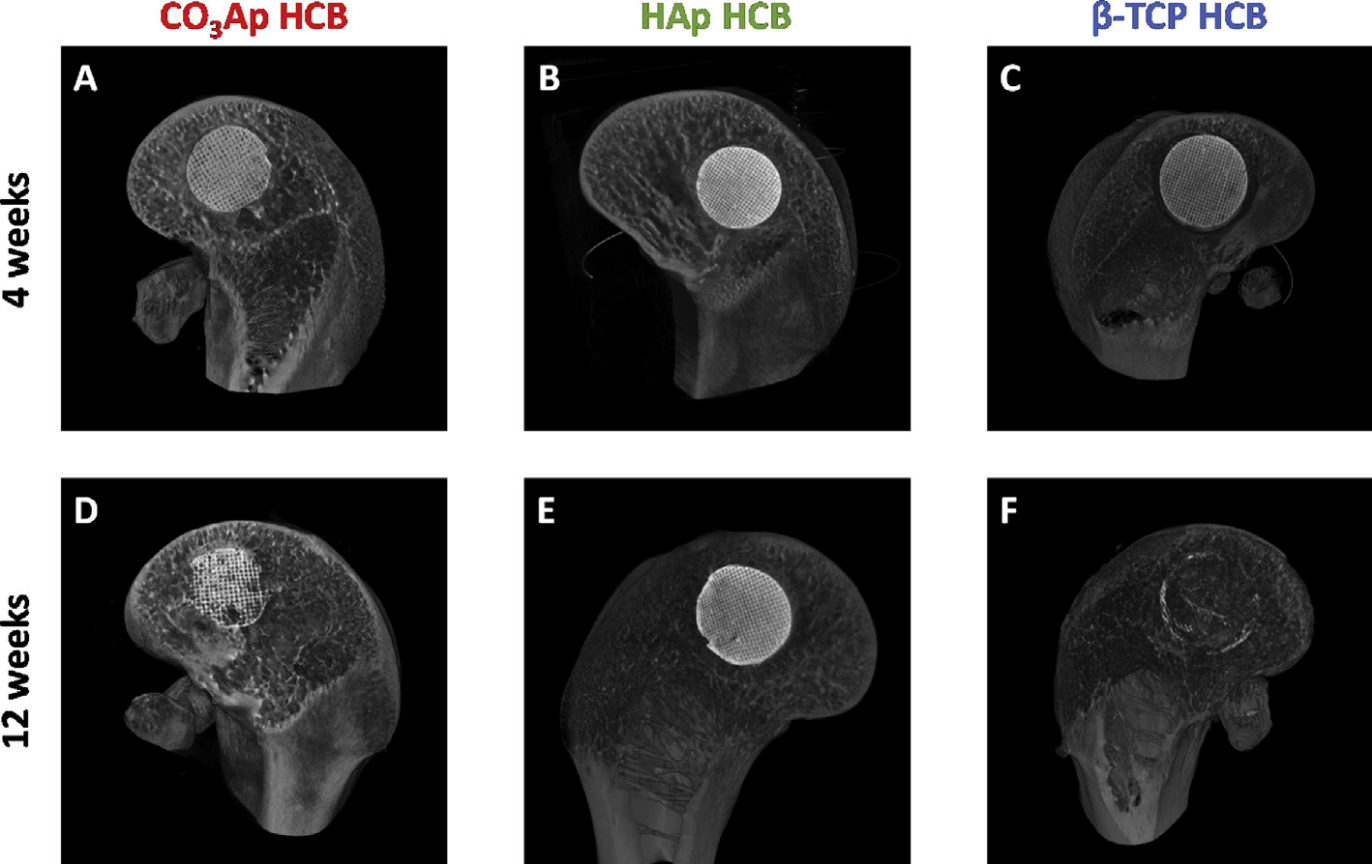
Examination of HCB resorption *in vivo*. μ-CT images of bone defects at POW 4 and 12 for CO_3_Ap (A, D), HAp (B, E), and β-TCP (C, F) HCBs, respectively.
